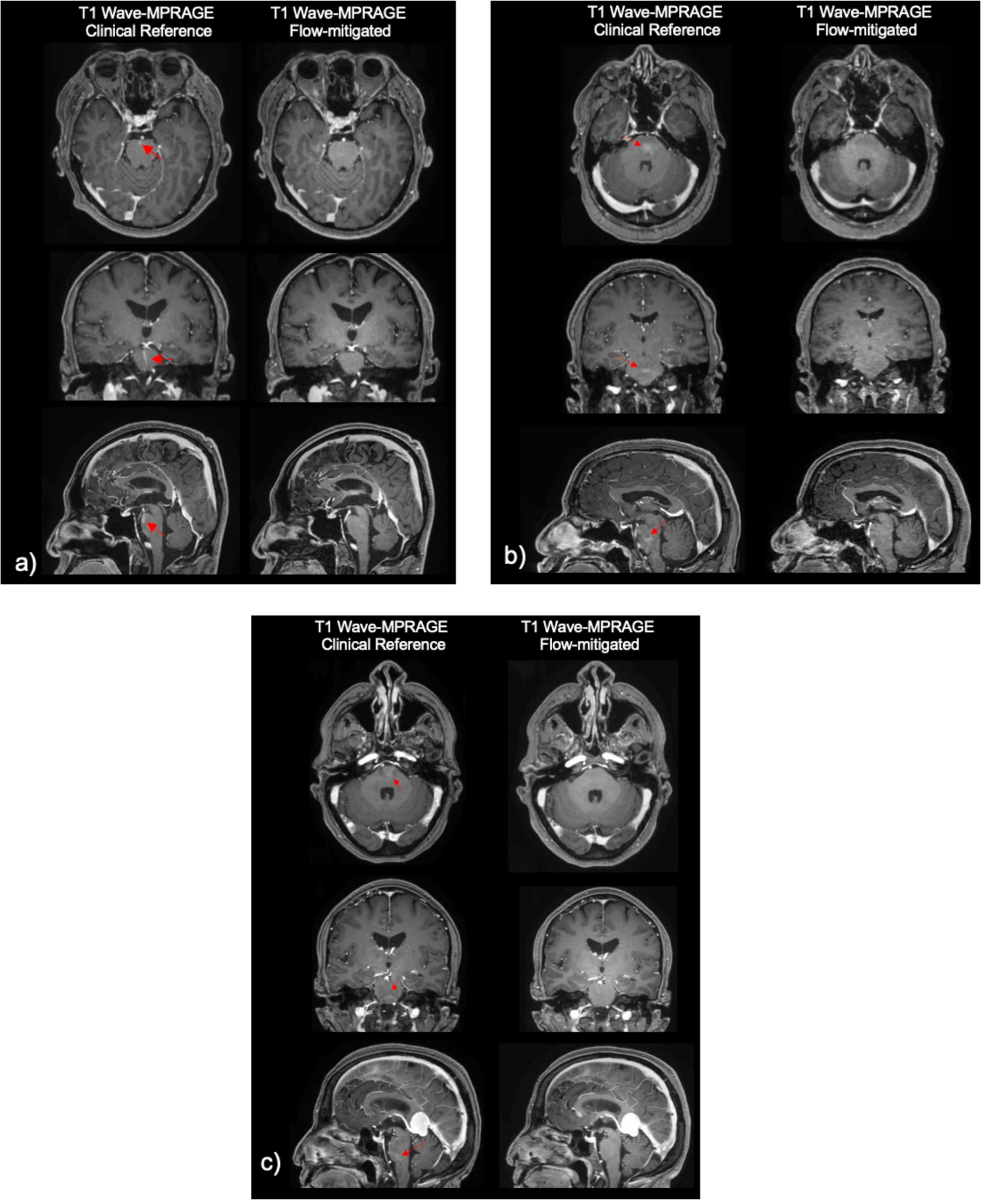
Representative examples of flow-related artifacts on conventional wave-controlled aliasing in parallel imaging (CAIPI) post-contrast T1-weighted magnetization-prepared rapid gradient-echo (MPRAGE) images that were reduced or eliminated on the flow-mitigated same sequence. Arrows point to the enhancing pseudo-lesions visible on multiplanar reformats of the conventional images. The abnormal enhancement was artifactual and corrected on the flow-mitigated Wave-MPRAGE images. Patient 1 (a): vascular pseudo-enhancement due to flow-related artifact in the ventromedian pons. The pseudo-enhancement is not seen on the flow-mitigated images. Patient 2 (b): an enhancing pseudo-lesion in the inferior pons. The flow-related pseudo-enhancement is not seen on the flow-mitigated images. Patient 3 (c): area of apparent enhancement on the conventional Wave-MPRAGE images mimics the appearance of an enhancing lesion in the pons, likely arising from flow in the basilar artery/venous plexus. No abnormal enhancement was seen in the pons on the flow-mitigated images
